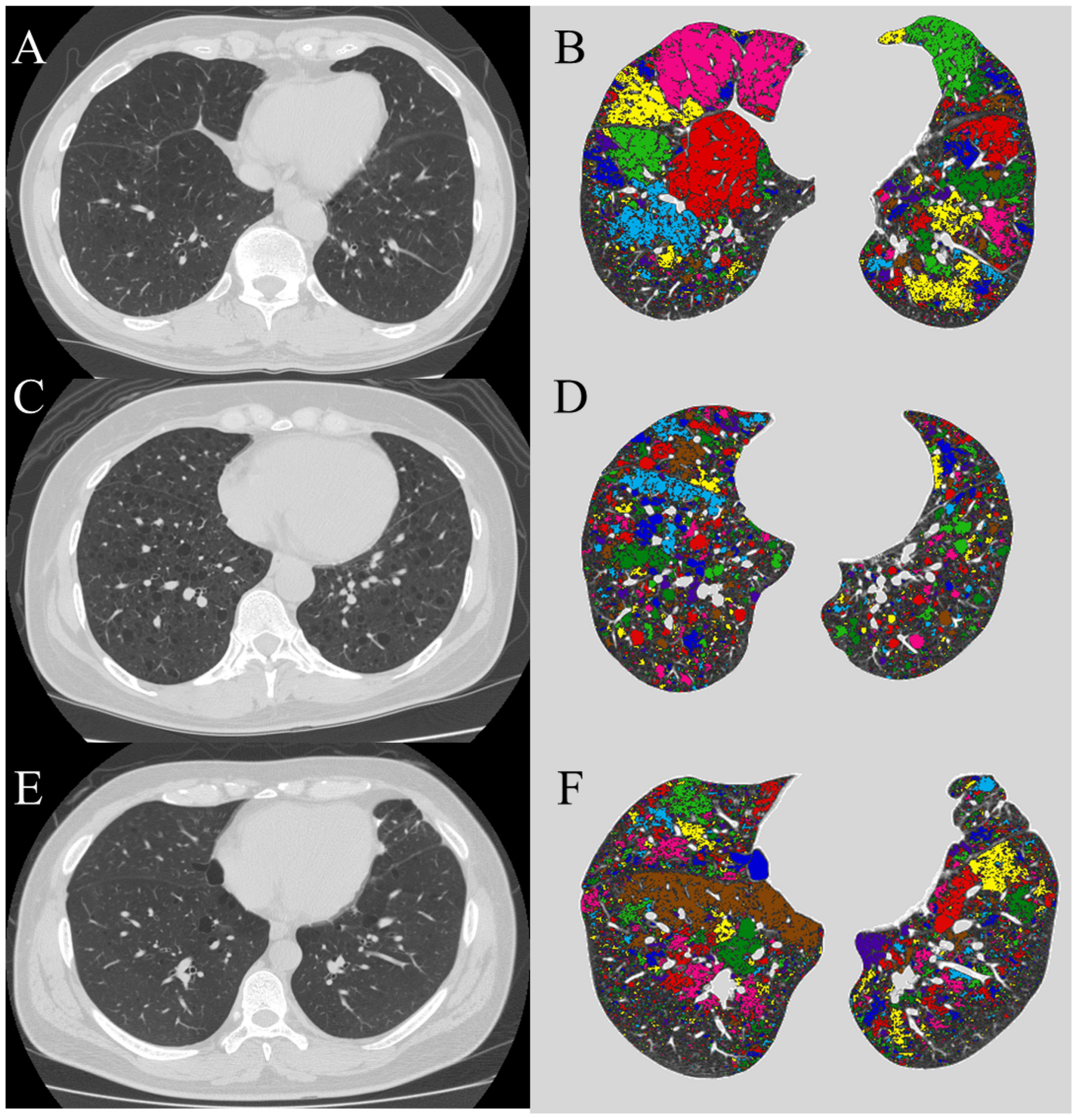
Analysis of LAA clusters on CT images. Original CT image of the lower lung field, and the same image as in original image but the individual cluster comprising contiguous LAA is shown in contrasting colors in a representative patient with COPD (A and B), LAM (C and D) and BHDS (E and F). The lung field was identified from the rest of the image, and the lumina of airways were excluded. In COPD, LAA also exists in seemingly normal region, and contiguous LAA regions (colored area) fuse and become large. On the other hand, in LAM and BHDS, LAAs are few in seemingly normal areas, and the contiguous LAA regions (colored area) seems to be independent. BHDS: Birt-Hogg-Dubé syndrome; COPD: chronic obstructive pulmonary disease; LAA: low attenuation areas; LAM: lymphangioleiomyomatosis.
